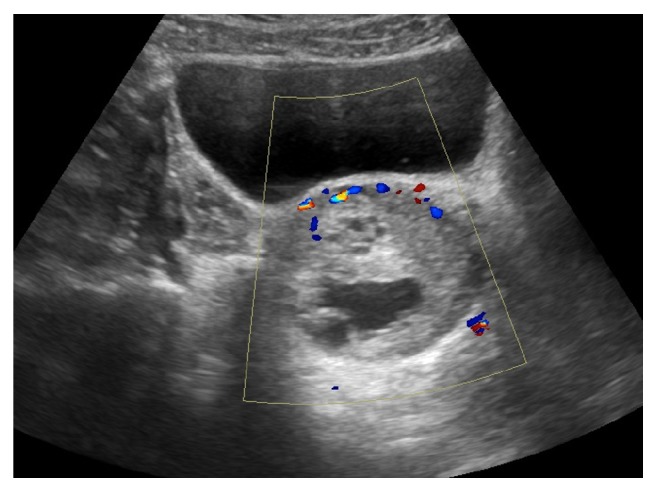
Ultrasound image of ovarian lesion.
